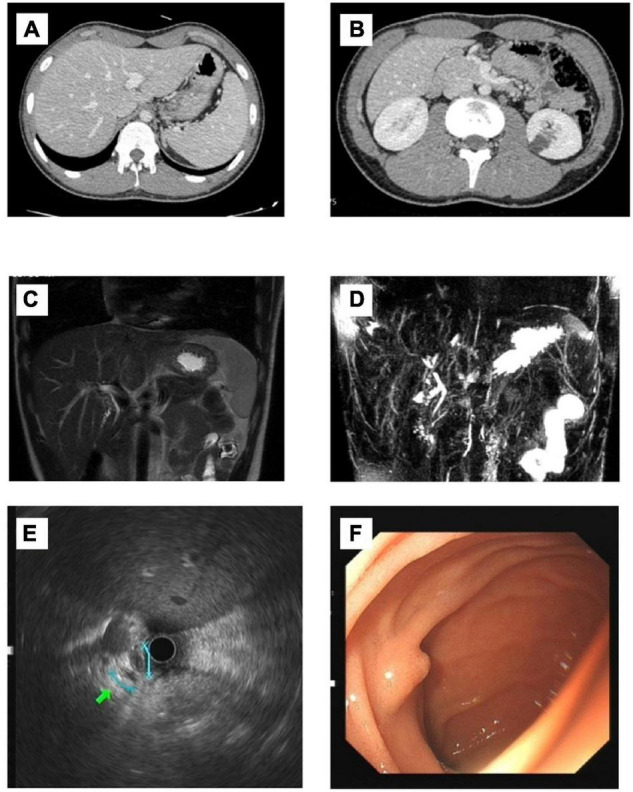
Imaging findings of the patient. (A,B) CT: No pathological findings were found. No dilatation of intrahepatic and external bile ducts was noted. There was no thickening or enhancement of extrahepatic bile ducts. The gallbladder is collapsed without bile filling; (C,D) MRCP: Possible stenosis at the beginning of the common hepatic duct. (E) Endoscopic ultrasonography: The extrahepatic bile duct was fine without dilation, and no definite obstruction was observed. (F) Gastroscopy: The size and morphology of the duodenal papilla were normal.
